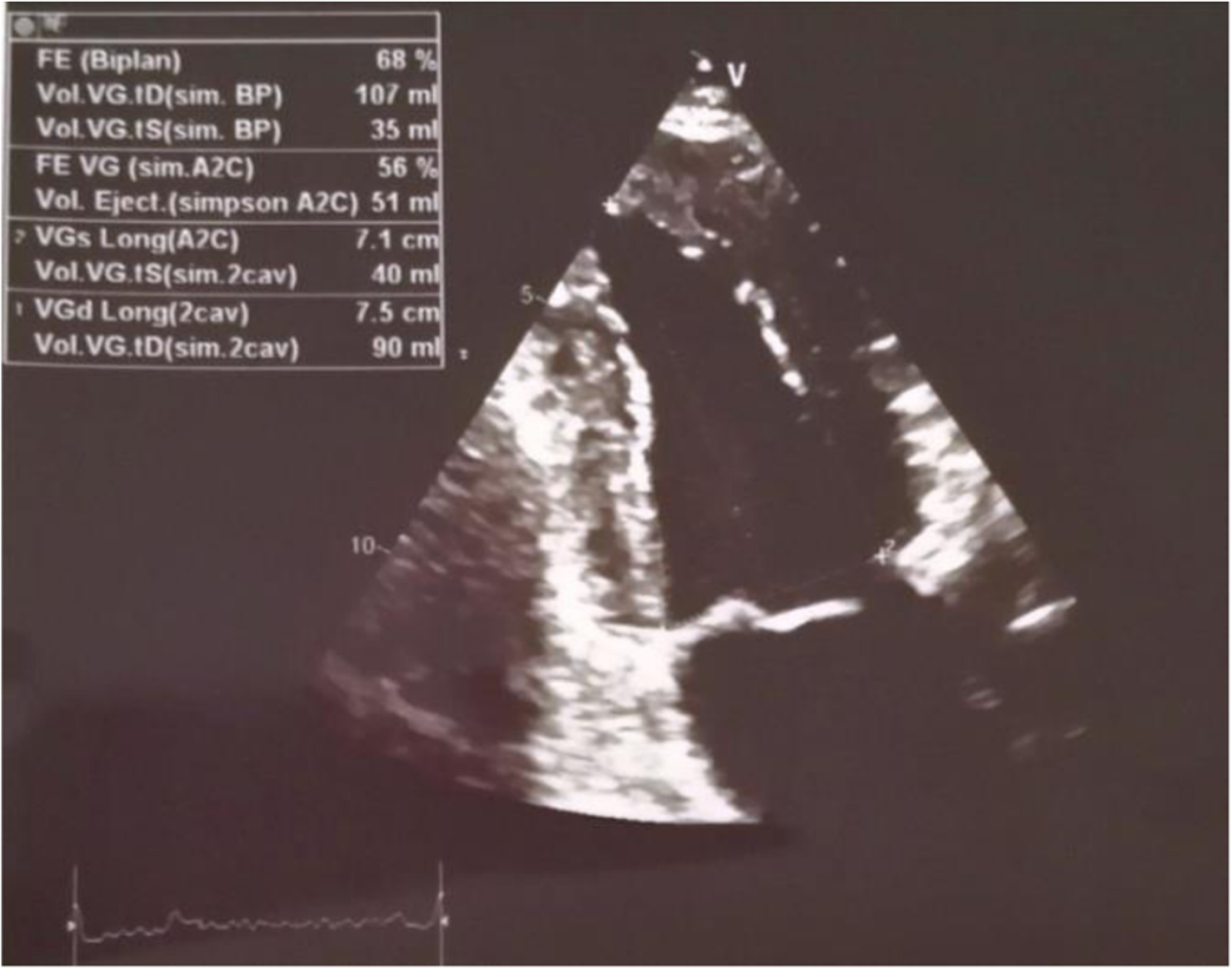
Transthoracic echocardiographic image showing the normal left ventricular size and function
